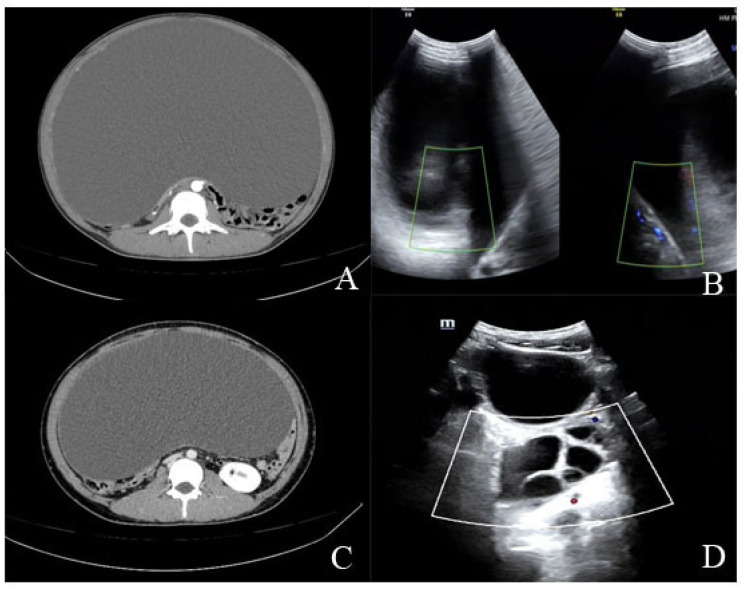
(A,B) Sister 1 preoperative whole-abdominal CT and color ultrasound. (C,D) Sister 2 preoperative whole-abdominal CT and color ultrasound.
